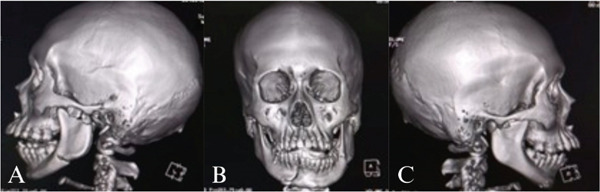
(A) CT showing medially displaced left condylar and angle of mandible fracture. (B) Symphysis fracture line. (C) The right neck condylar fracture.
